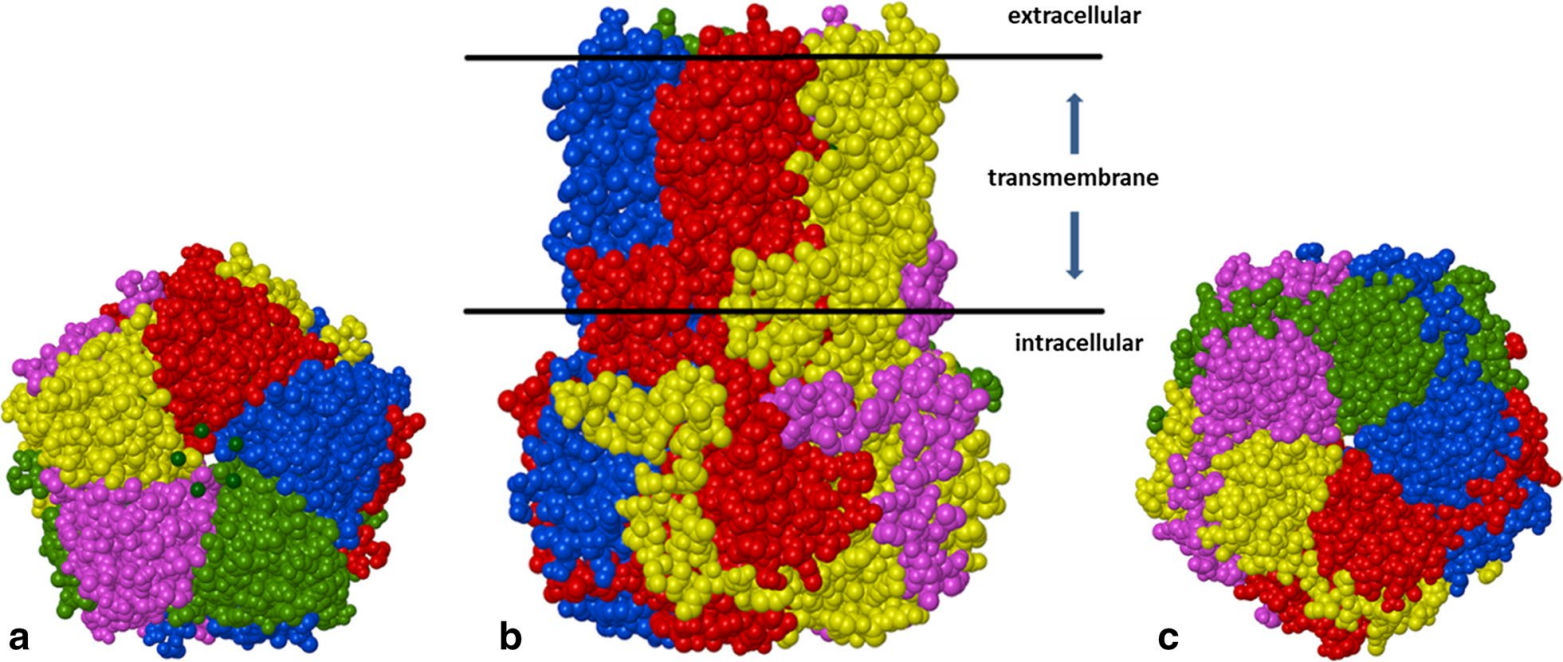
X-ray structure of bestrophin-1 from *Gallus gallus* [9, 10] forming a calcium-activated chloride transmembrane channel (CaCC) composed of five identical subunits and containing a single long pore along the axis of symmetry. a Top view (extracellular side), b side view, c bottom view (cytosol side, channel aperture). The CaCC is shown in Corey–Pauling–Koltun (CPK) representation, dark green spheres—chloride ions (A), orange spheres—potassium ions (not visible), blue spheres—calcium ions (not visible)
